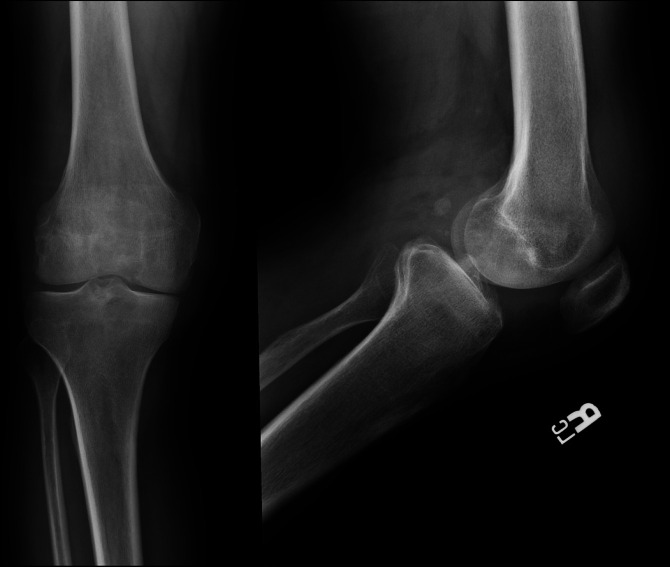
AP (left) and lateral (right) preoperative radiographs obtained 1 month before the index total knee arthroplasty procedure and roughly 1 year after the patient's initial development of the skin lesion demonstrating rapidly progressive arthritis.
